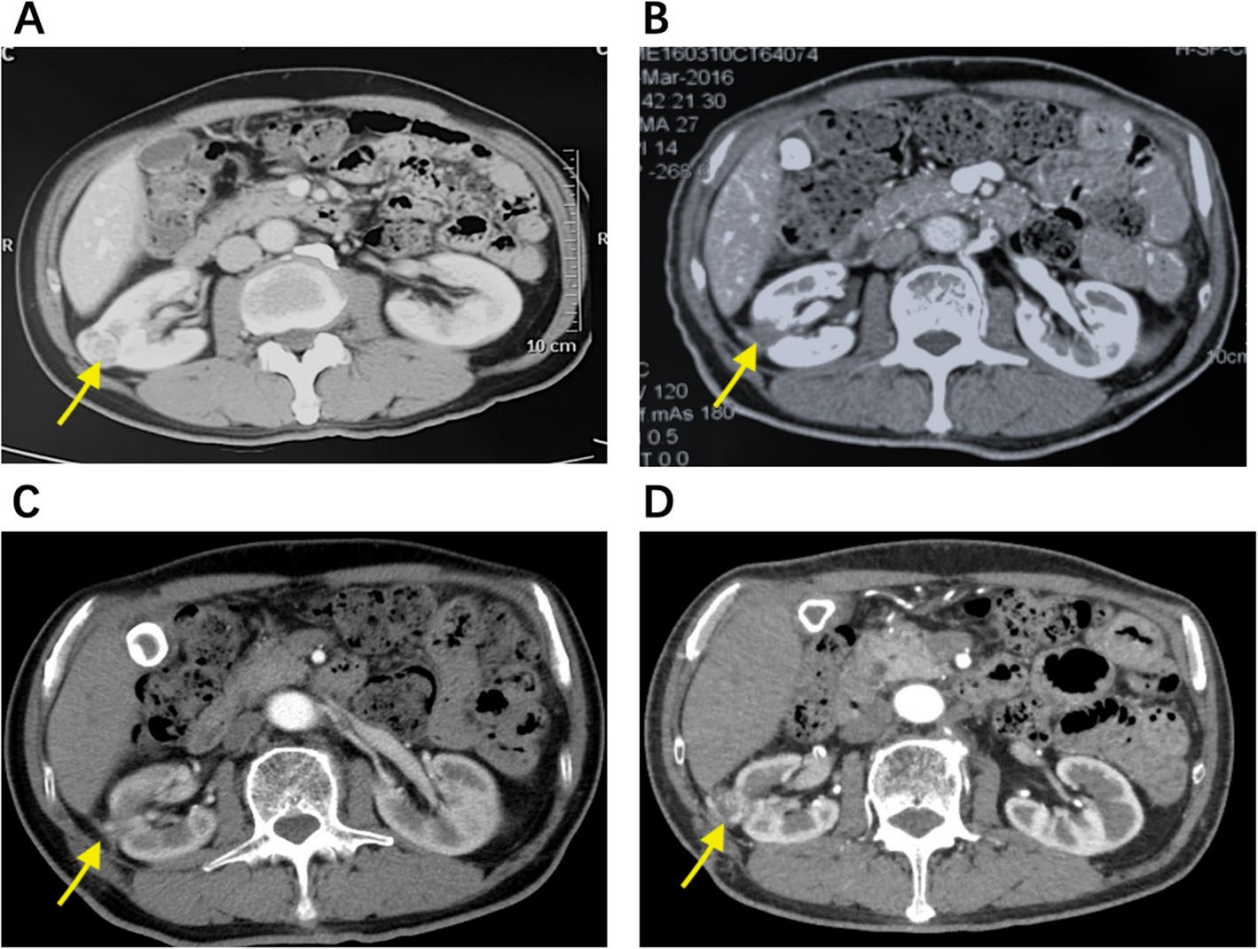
Ablation and recurrence after PCA (shown by arrows). A Contrast-enhanced CT before cryoablation, showing nonuniform enhancement of tumor. B One week after ablation, the tumor showed no enhancement, indicating that the tumor has been completely ablated. C Twenty-four months after the operation, the tumor still has no enhancement, indicating no recurrence. D Sixty-one months after the ablation, a tumor appeared at the original location with enhancement, indicating recurrence
